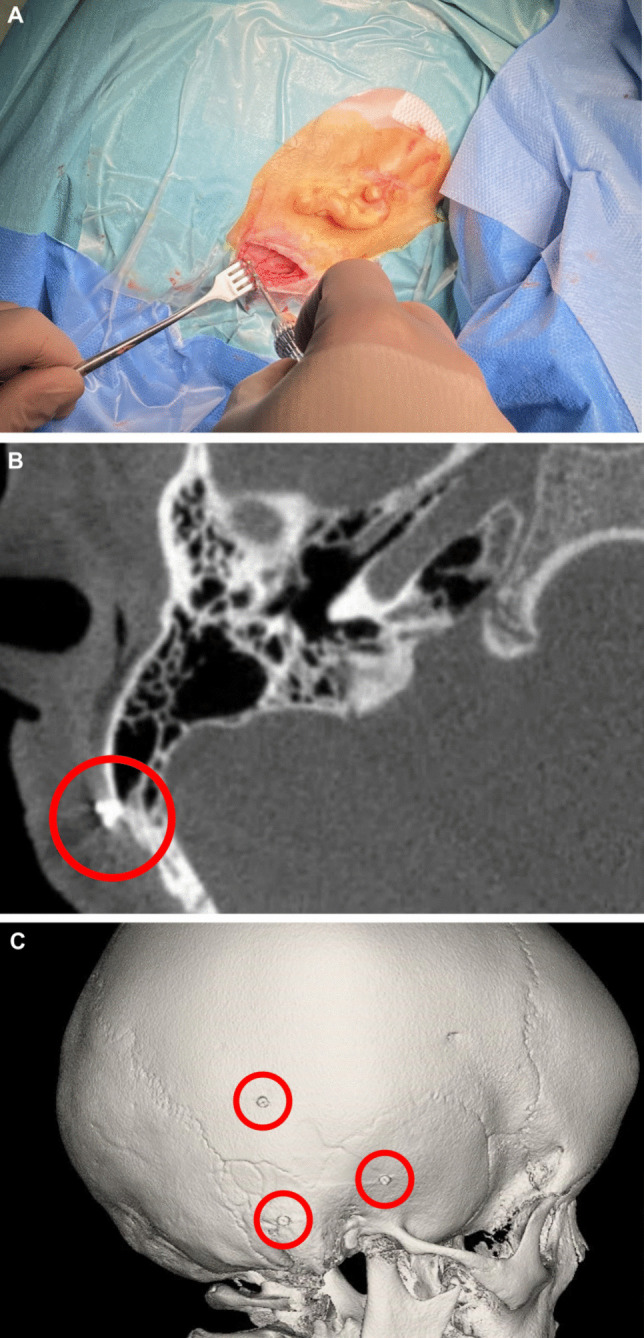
Implantation of screws for electromagnetic navigation A) Surgical incision and implantation of self-cutting screws. B) Axial plane from an intraoperative CBCT scan showing an implanted screw. C) 3D reconstruction from the CBCT scan displaying the locations of all three screws
